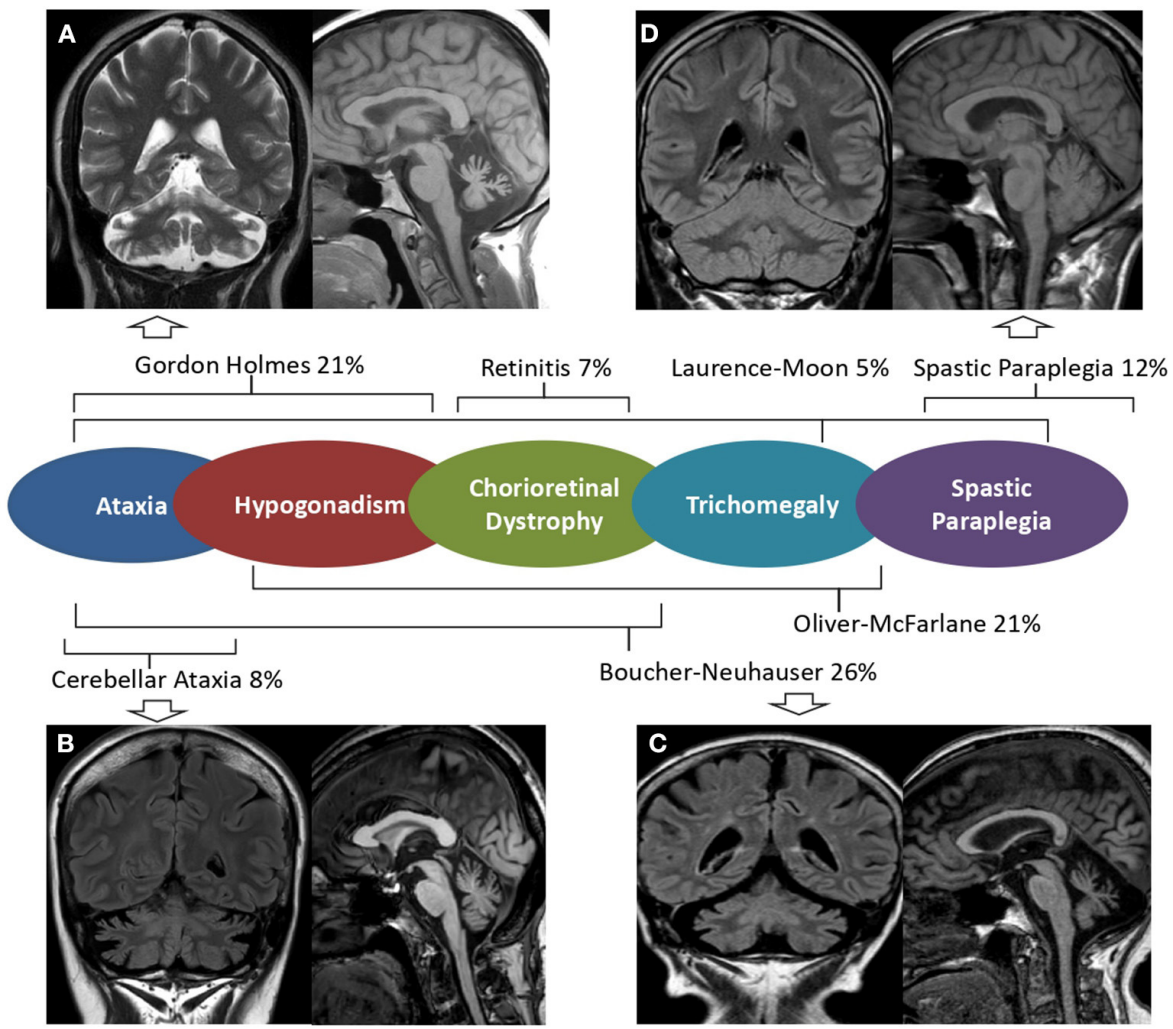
Brain MRI in *PNLA6*-associated phenotypes. Mid-sagittal T1 weighted and coronal FLAIR or T2 w brain MRI images of patients with *PNPLA6*-associated clinical phenotypes. Severe cerebellar atrophy and slightly hyperintense dentate nuclei are shown in patients with Gordon Holmes syndrome [(A), Patient 4], late-onset cerebellar ataxia [(B), Patient 7], and Boucher Neuhauser syndrome [(C), Patient 3]. No MRI abnormalities are detectable in patients presenting Spastic Paraplegia [(D), Patient 1]. A central scheme summarizes the frequency of the syndromic diagnoses reported in the literature.
